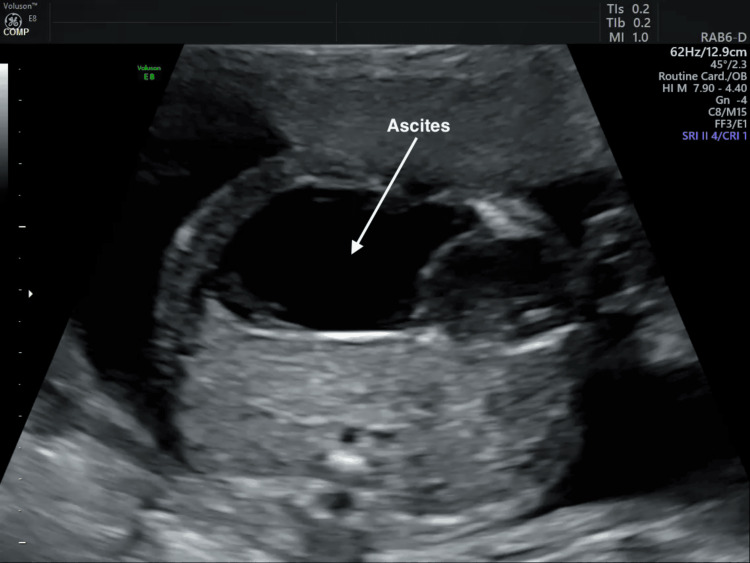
Prenatal ultrasound showing massive fetal ascites Transabdominal ultrasound at 23 weeks' gestation showing a markedly distended fetal abdomen with free intra-abdominal fluid consistent with massive fetal ascites.
